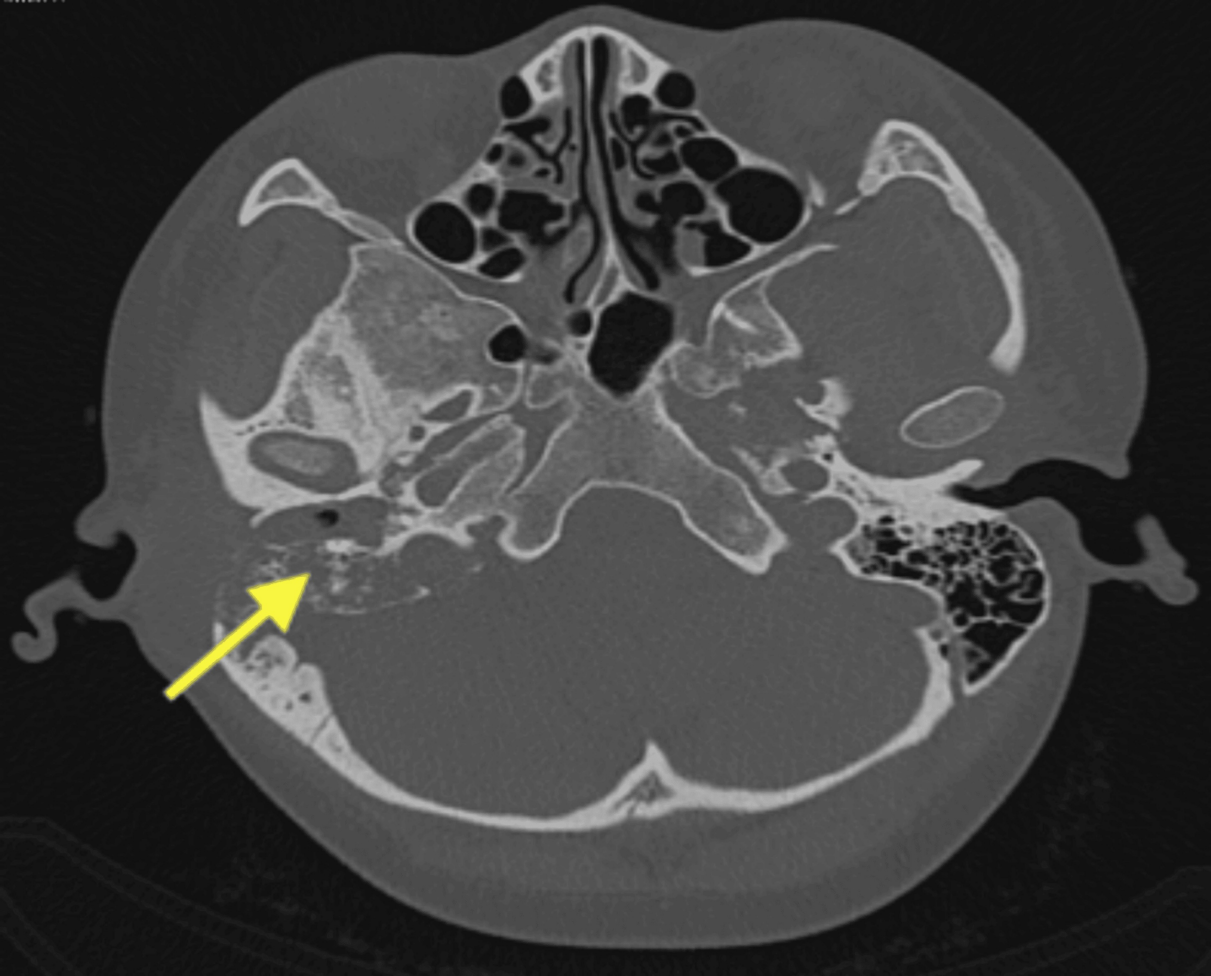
HRCT temporal bone scan showed right acute otitis media and mastoiditis with extensive bony erosion HRCT, high-resolution CT
